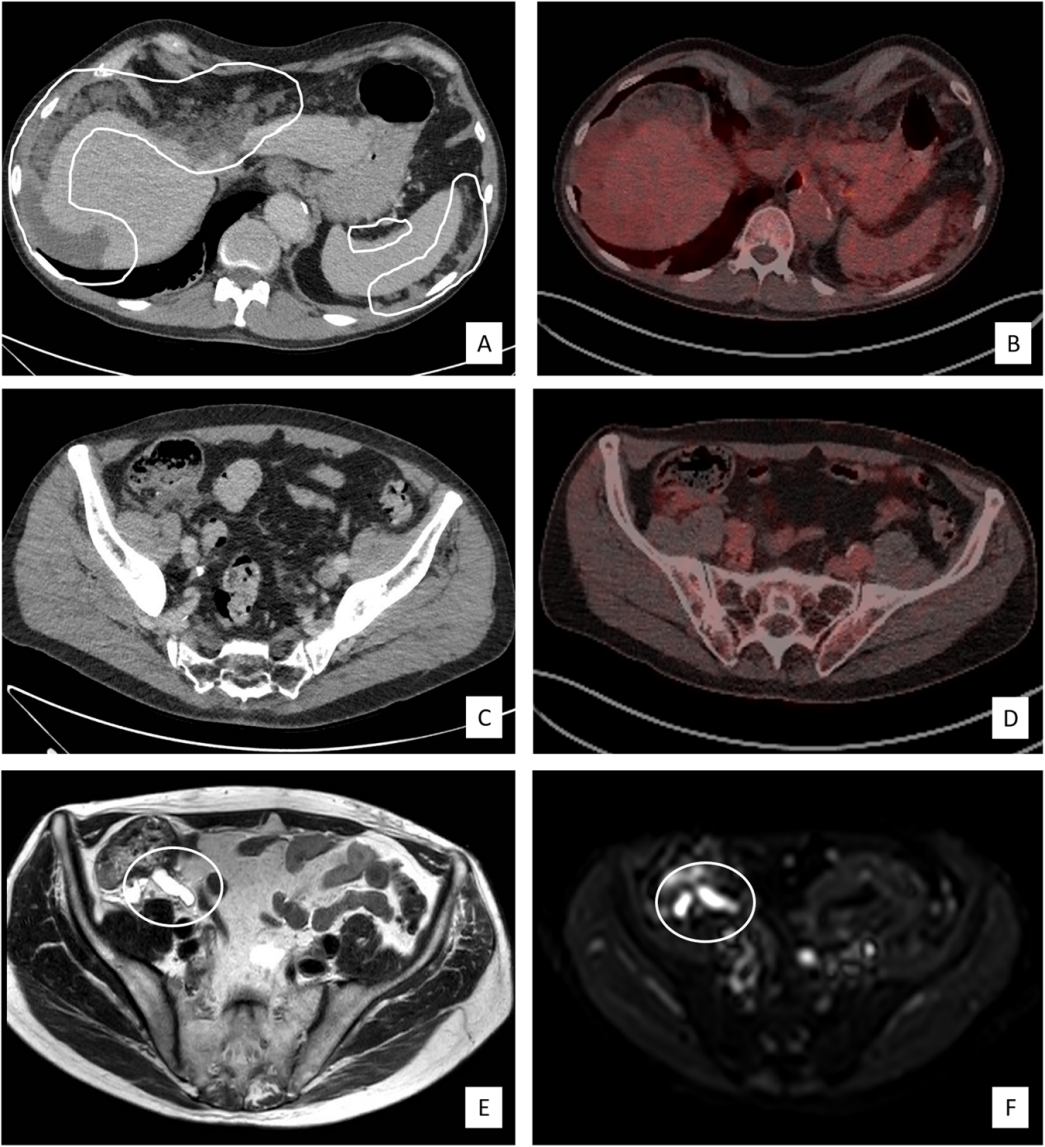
Patient (#26, see Supplement 1) diagnosed on CT with extensive peritoneal metastases in the upper abdomen around the liver and spleen (A); the peritoneal lesions showed only mild FDG uptake on corresponding PET/CT (B). No primary tumour was reported on CT (C) or FDG-PET/CT (D); no abnormalities were reported in the region of the appendix (though, in retrospect, the appendix may have been visible on CT). On T2-weighted MRI, a pathologically thickened appendix filled with fluid (mucine) was identified, which was also clearly visible on the low b-value (b0) diffusion-weighted images (circles in E, F), which led to the correct final diagnosis of a mucinous adenocarcinoma of the appendix as the underlying primary tumour
